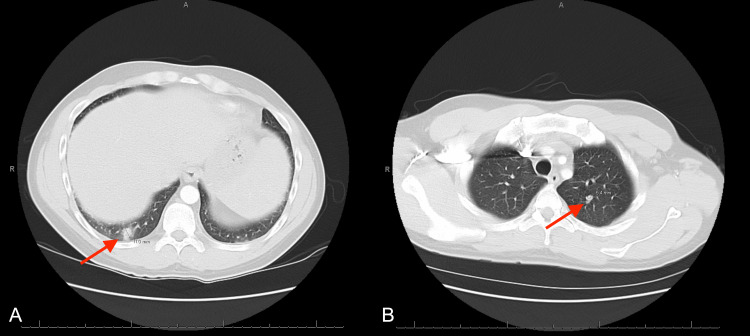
CT pulmonary angiogram (A) Lung nodule in the right lower lobe measuring 11 mm. (B) Lung nodule in the left upper lobe measuring 5.4 mm.
